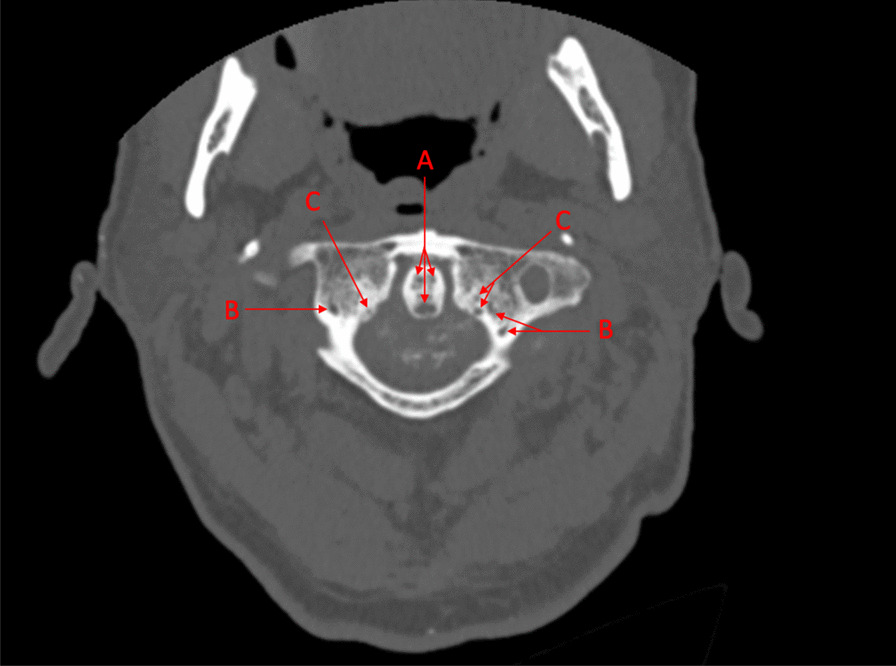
Transverse computed tomography of atlantoaxial joint with noticeable involvement of the odontoid process of axis (a), the lateral masses of atlas (b), the tubercles of atlas (c) for the transverse ligament, and the transverse ligament itself
